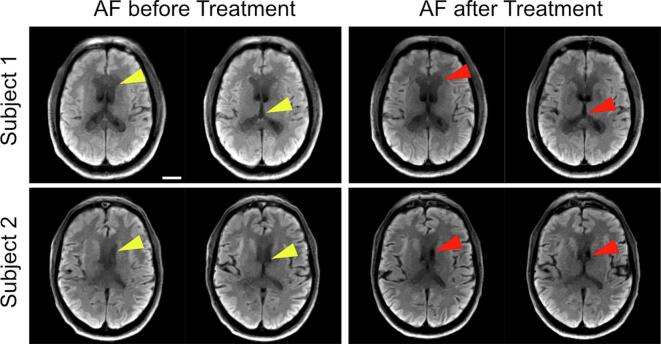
Diffusion-weighted imaging (DWI) b = 500 s/mm^2^ in subject #1 and #2 before and after treatment. At b = 500 s/mm^2^, the signal suppression is incomplete, potentially providing insights into CSF dynamics within the ventricular system. Signal voids in the ventricles (yellow arrowheads, before treatment) are more prominent after treatment (indicated by red arrowheads). Scale bar 2 cm.
